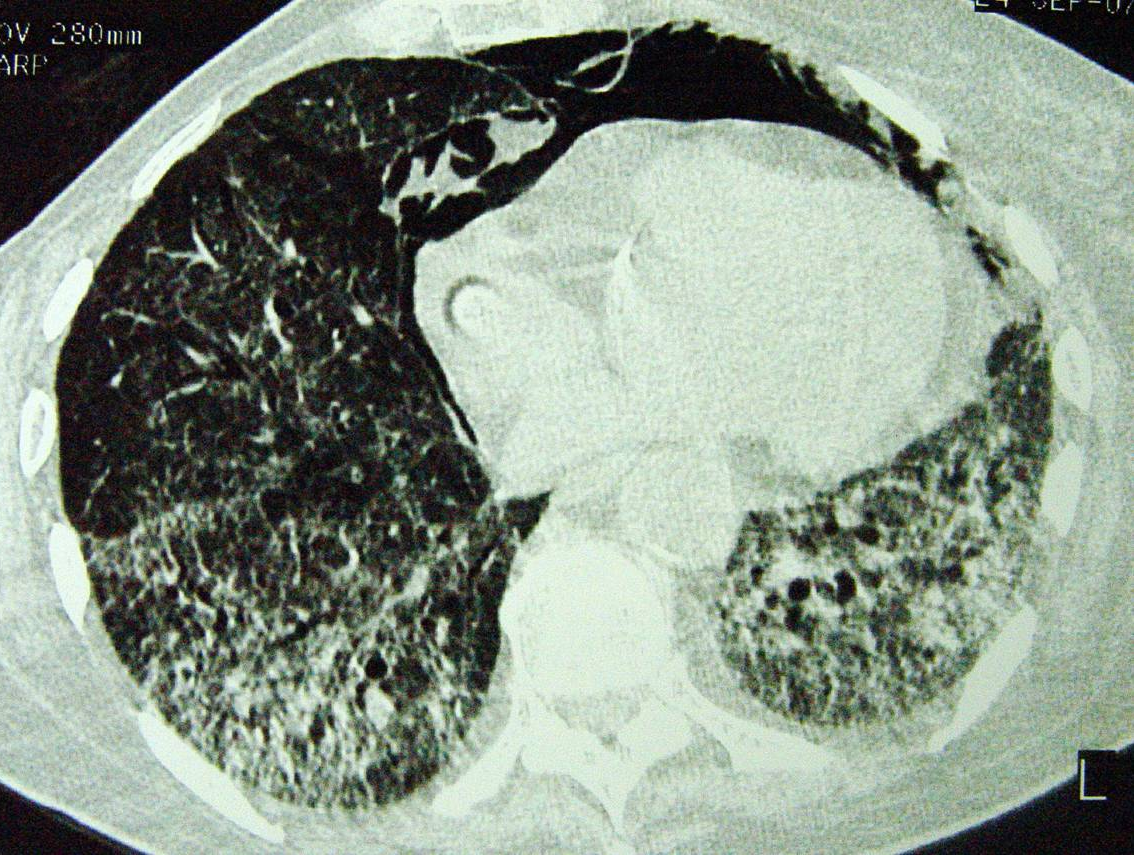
Computed tomography demonstrating organized consolidations with air bronchogram at the lung bases and air in the mediastinum.
